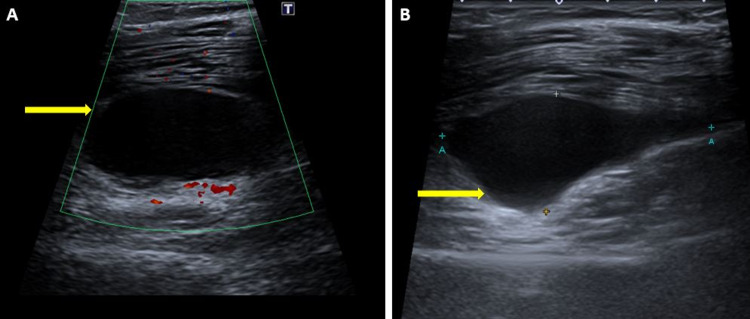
Ultrasound of the right thigh demonstrating a well-defined cystic lesion (yellow arrows) within the tendon of semimembranosus with no internal vascularity.
